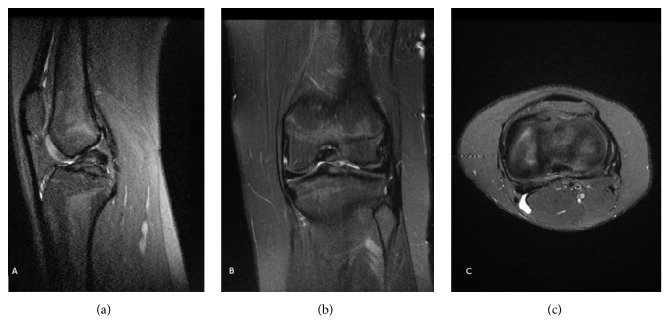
MRI (sagittal (a), coronal (b), and transverse (c) views) of the left knee of the patient from Figure 6 showing the same findings as her right knee.
